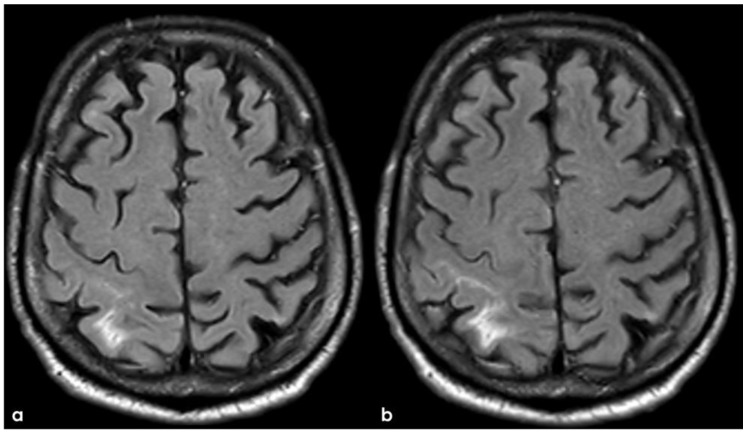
Axial FLAIR before and after contrast administration (panel (a) and (b), respectively), showing the spontaneous sulcal hyperintensity with increasing extension in a post-contrast image.
